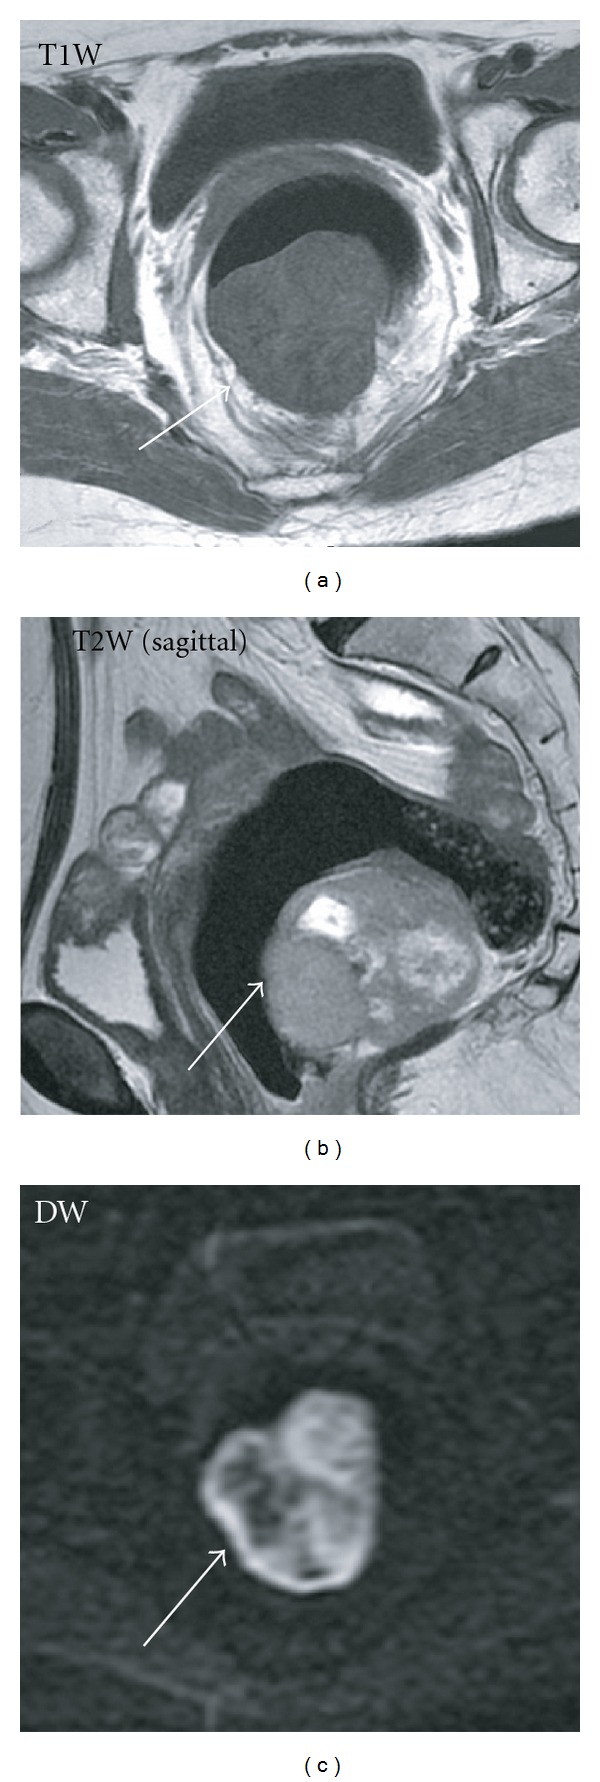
MRI imaging of tumor (T1-weighted (a), T2-weighted (b), and diffusion-weighted (c) images).
